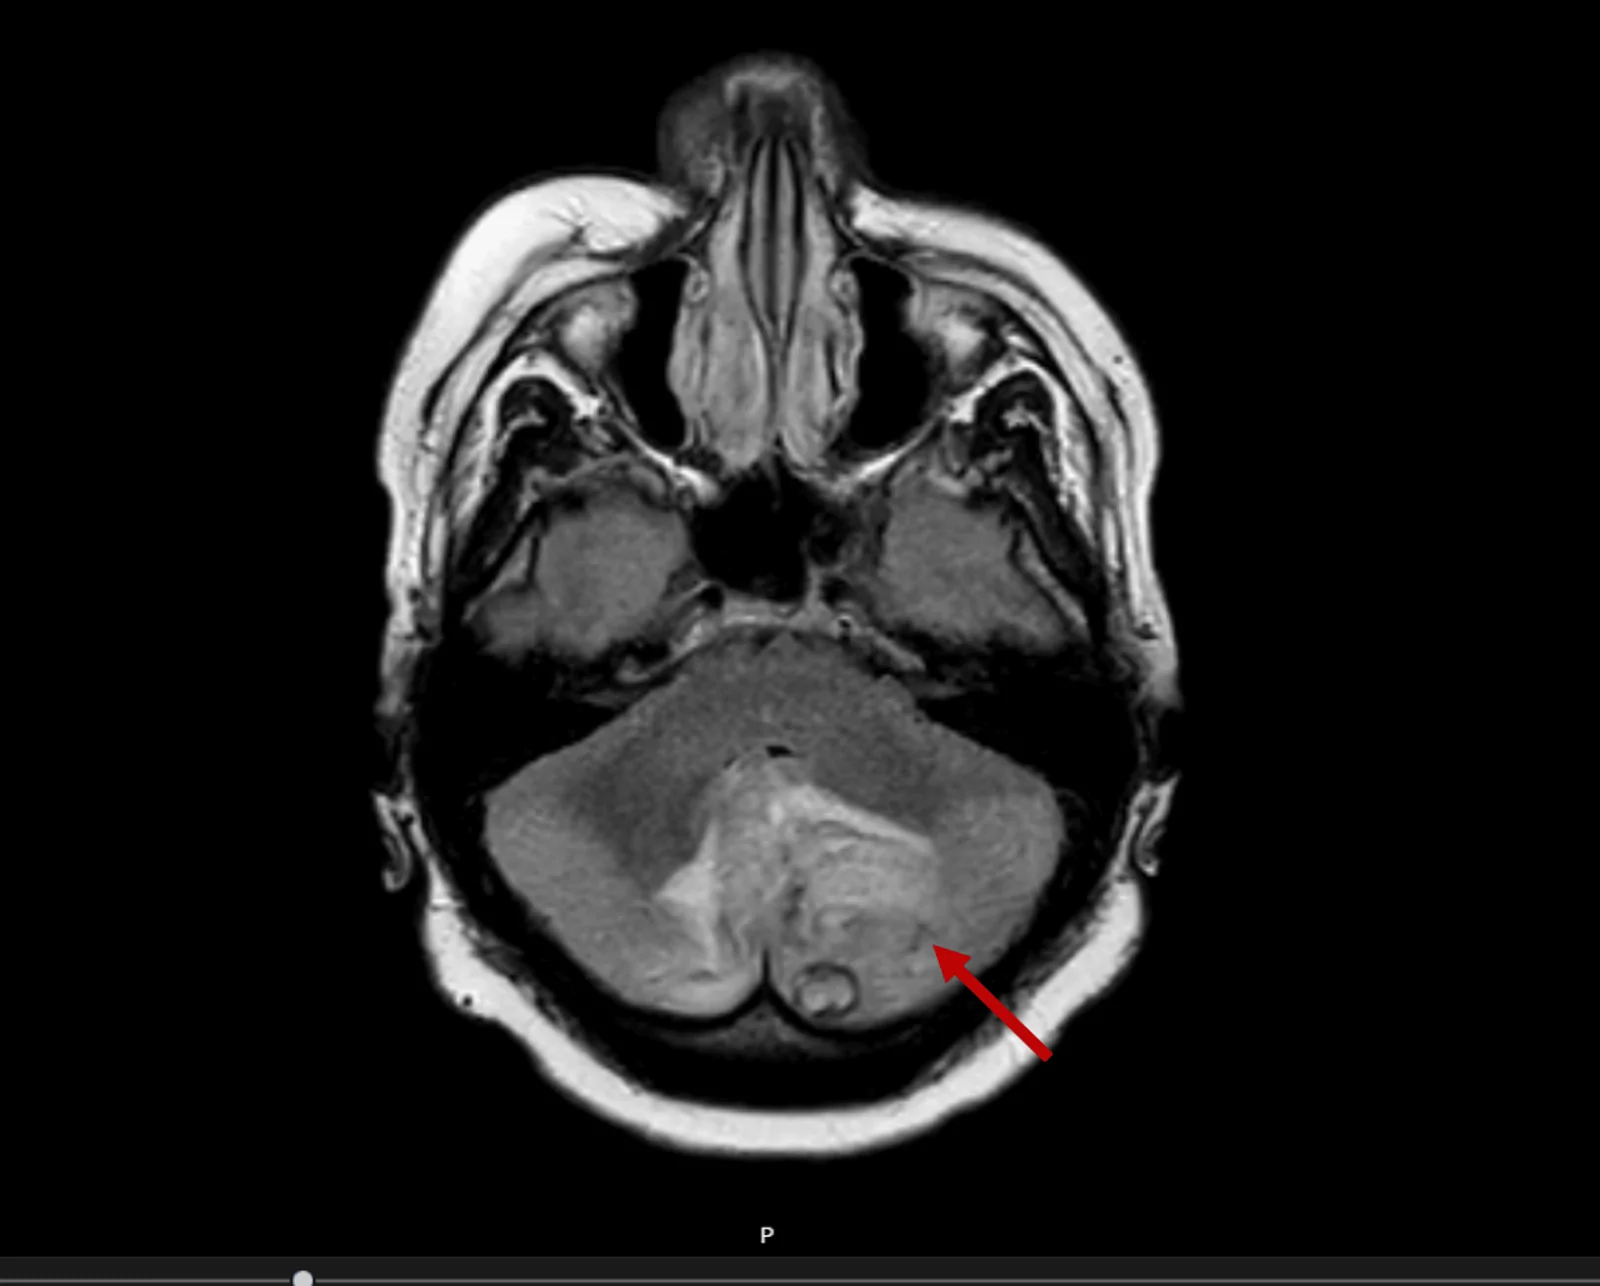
Axial MRI of the brain Left cerebellar haemorrhage (arrow).
